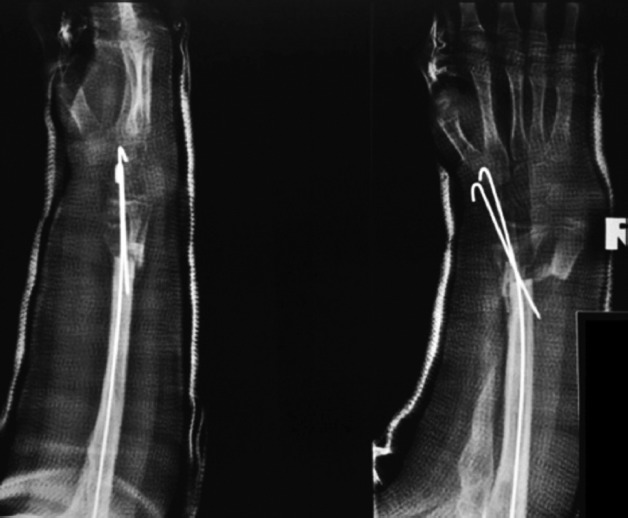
Immediate post-operative radiographs of the patient.
